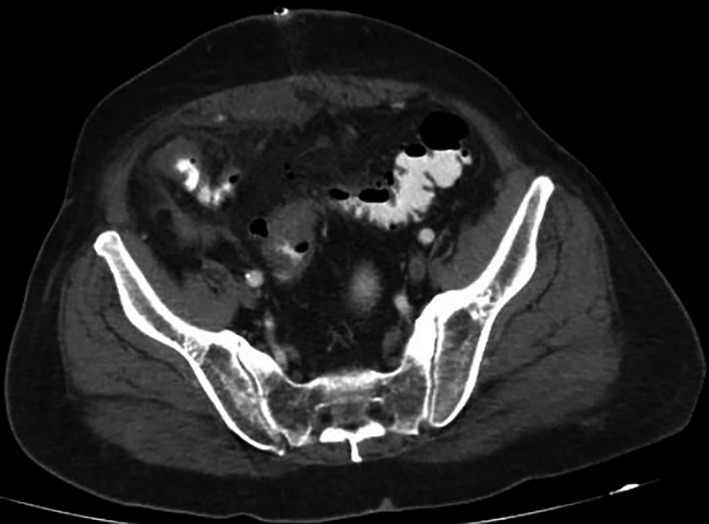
CT depicted extensive rectosigmoid wall thickening and pelvic extravasation of gas and fluid
